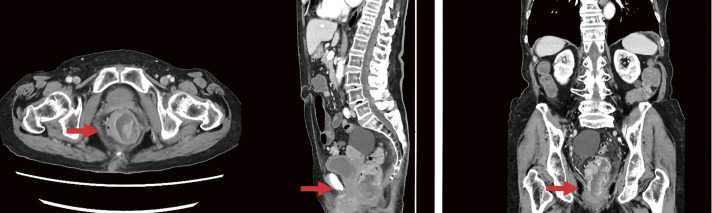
Computed tomography images. Multiple tumors on the left wall of the rectum below the peritoneal reflection protruding from the anus and two enlarged pararectal lymph nodes were observed, while no obvious distant metastasis was found.
